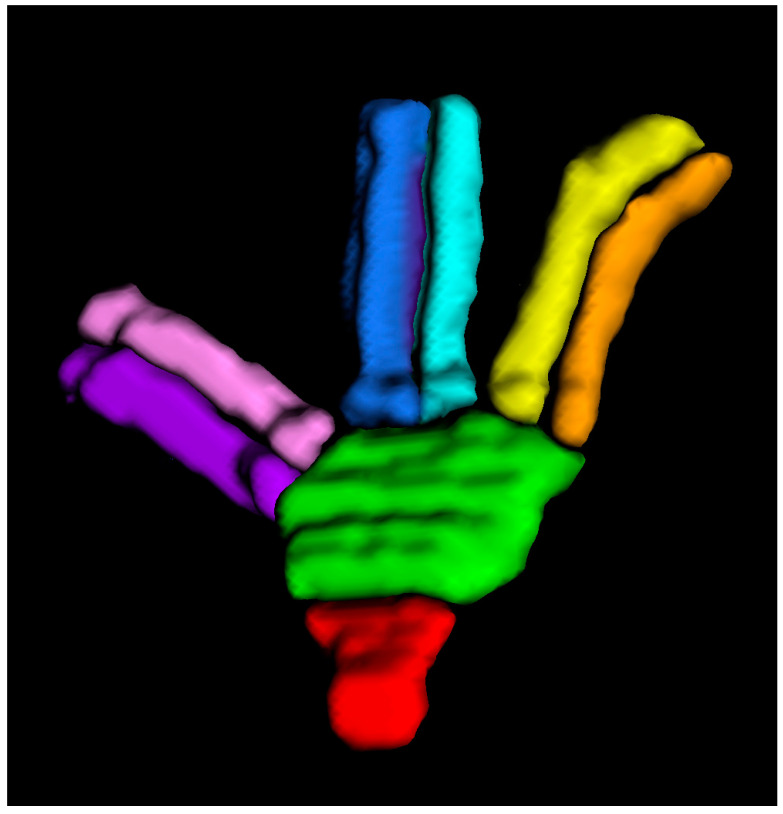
The 8 surfaces of Meckel’s cave that were rendered with ITK-SNAP software from CT scans. Red: trigeminal stem; green: Gasserian ganglion; orange: V1 medial; yellow: V1 lateral; light-blue: V2 medial; blue: V2 lateral; purple: V3 medial; violet: V3 lateral.
